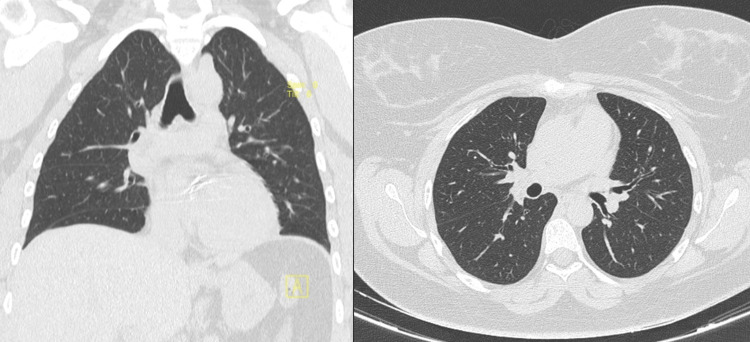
Chest CT scan showing no interstitial abnormalities, pleural effusions, or significant pulmonary pathology despite severe restrictive impairment CT: computed tomography
